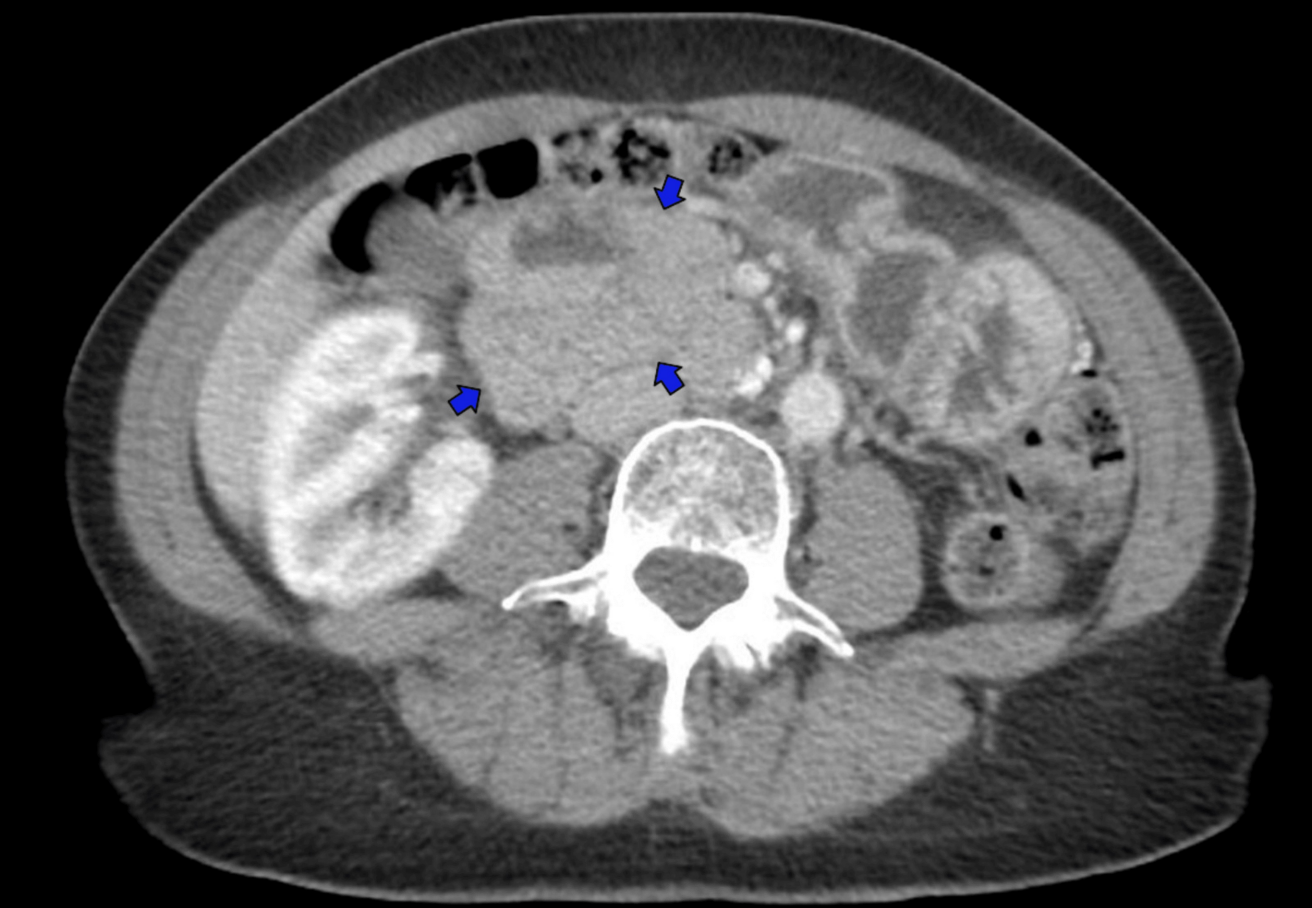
Axial CT of the abdomen and pelvis demonstrating a right upper quadrant hernia containing the small bowel (blue arrows)
